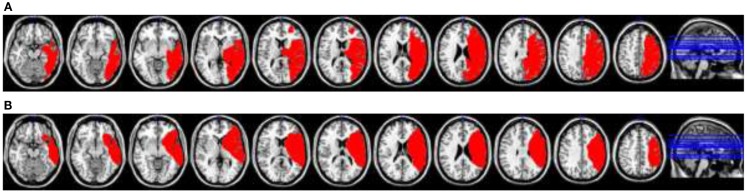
(A) Patient 1’s lesion reconstruction (in red) plotted from magnetic resonance imaging acquisitions onto a standard MRI-based template. (B) Patient 2’s lesion reconstruction (in red) plotted from magnetic resonance imaging acquisitions onto a standard MRI-based template.
